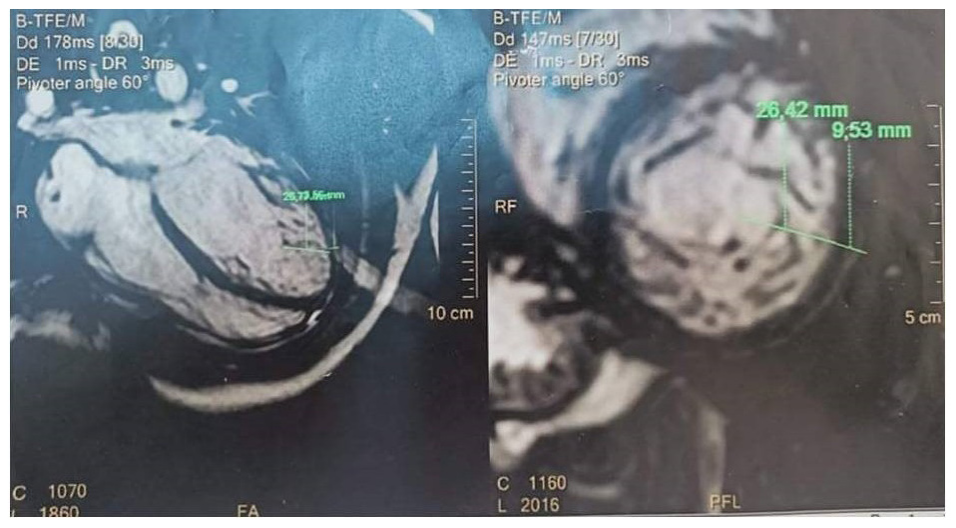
Cardiac magnetic resonance imaging showing non compacted trabeculation.
